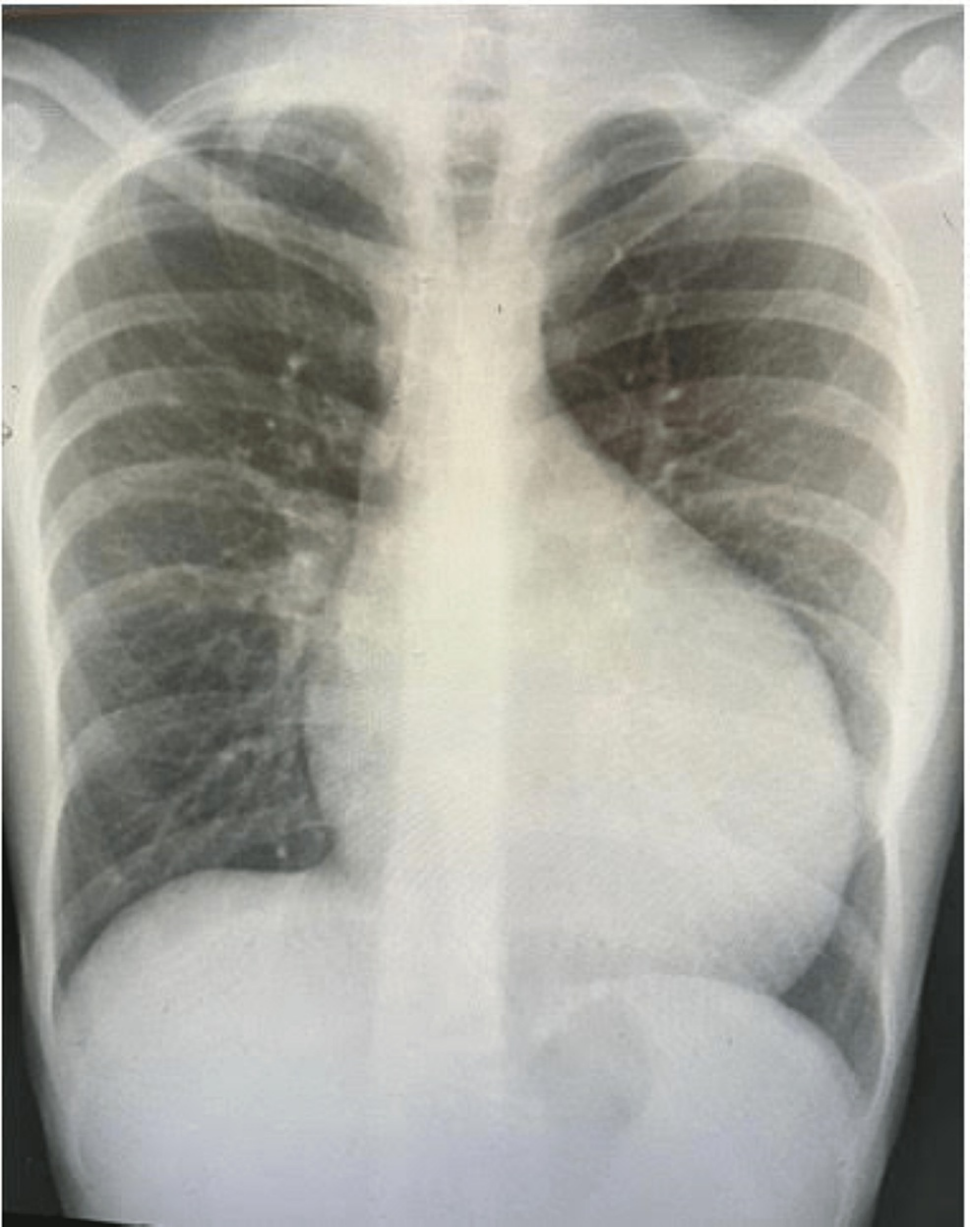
Posterior-anterior (PA) view of the chest X-ray showed an enlargement of the cardiac silhouette
